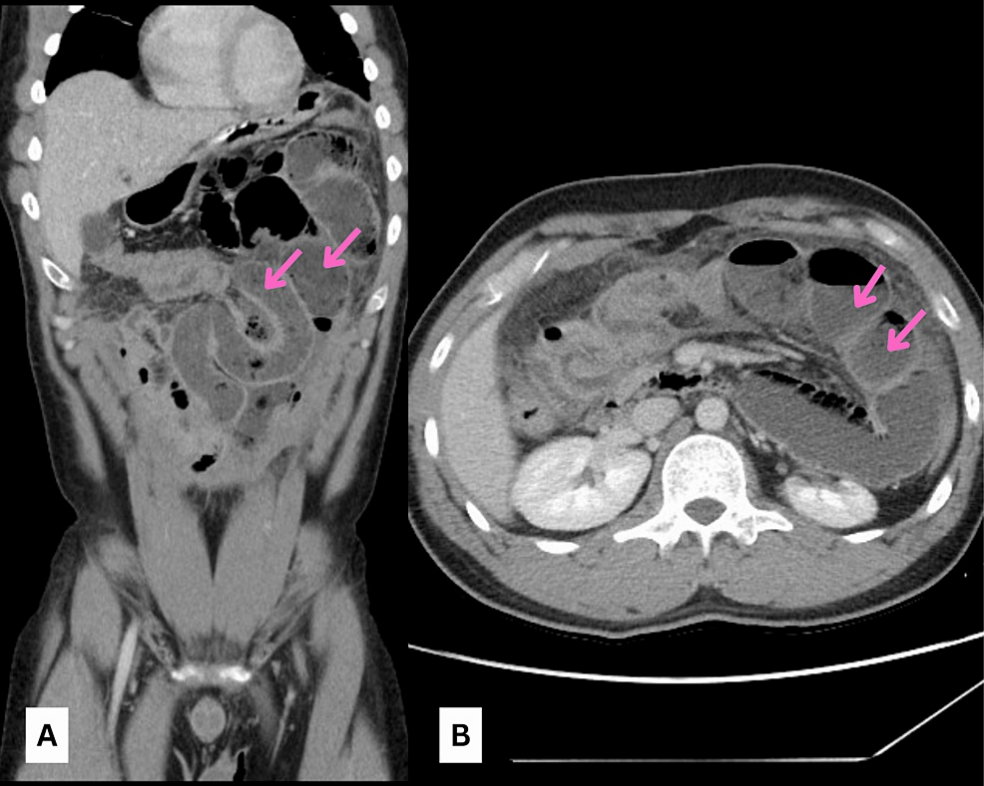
Abdominal CT scan images: (A) coronal view and (B) transverse view showing dilated jejunal loops (pink arrows). CT, computed tomography
